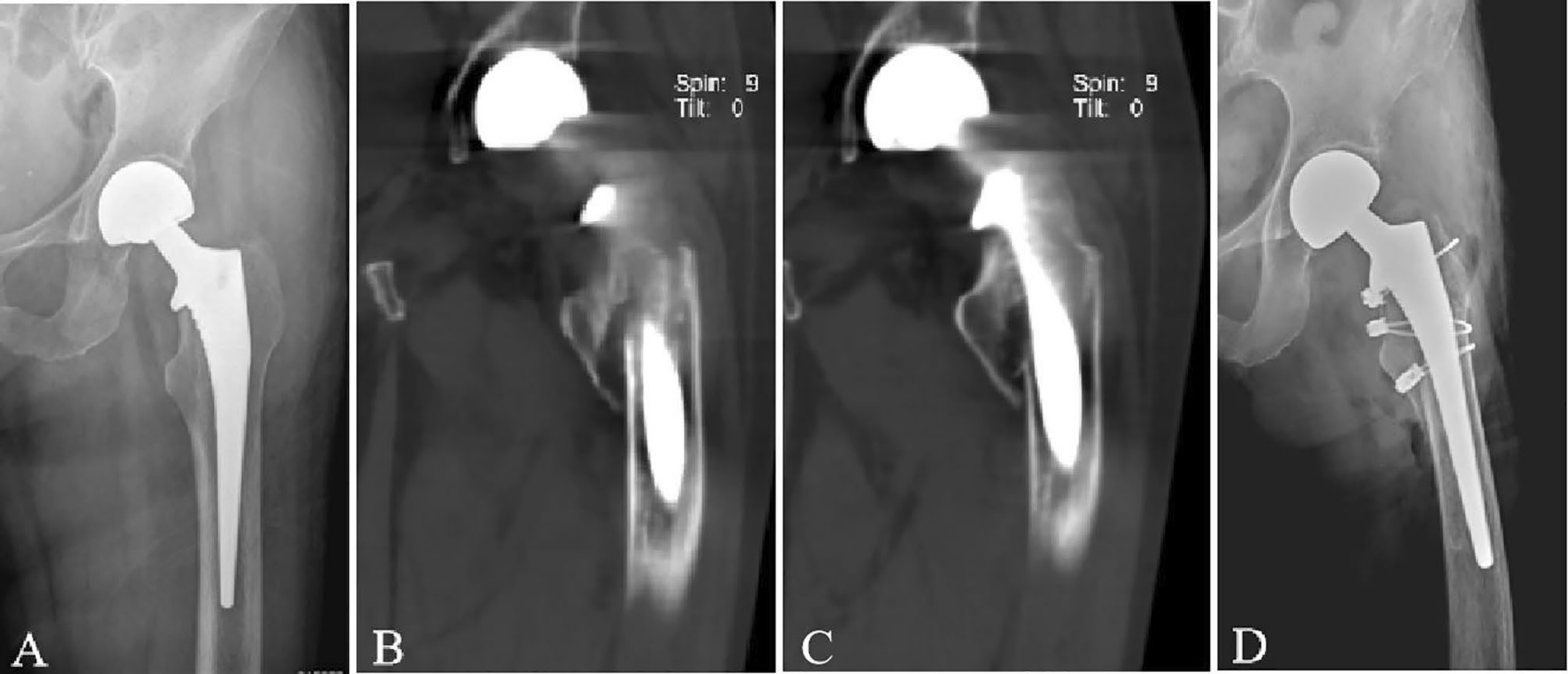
Case 19, an 88-year-old woman. (A) Anterior–posterior radiograph after hemi-arthroplasty. (B–C) Two coronal CT images show the periprosthetic fracture. (D) Anterior–posterior radiograph shows the surgical treatment of open reduction and internal fixation with cables.
